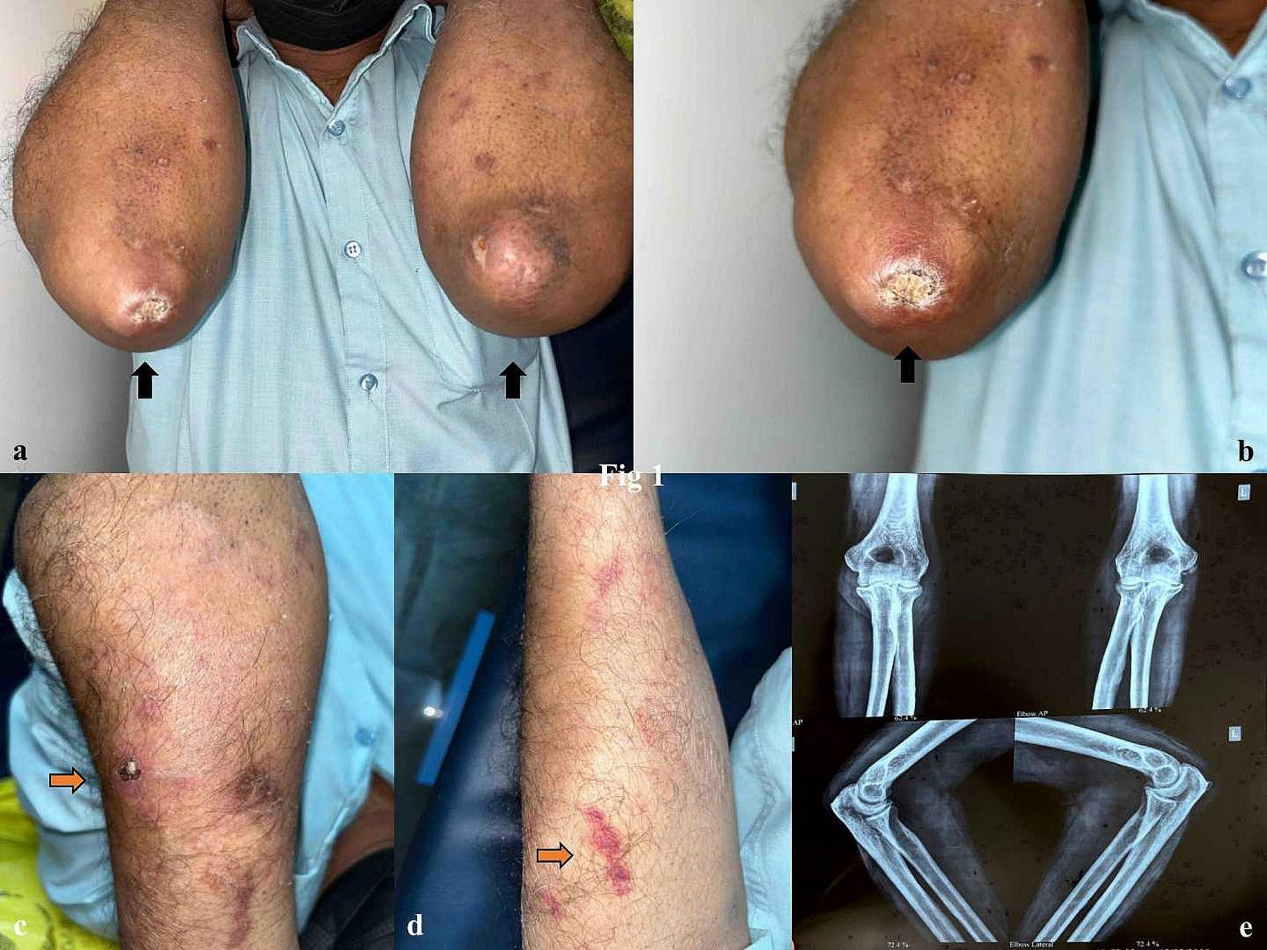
a,b,c,d: Swelling in the right elbow posterior aspect measuring 2 × 2 cm, firm to hard and non-tender. Another swelling in the left elbow is firm to hard with overlying reddish skin. There were a few purpuric lesions on the arms with superficial crusting. e: X-ray from B/L elbow shows inflammation with irregular fluid collection into the posterior aspect of the right elbow in the subcutaneous plane and irregular erosion of the olecranon
